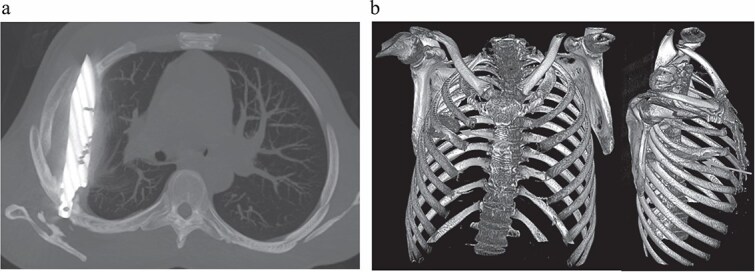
(a) CT chest maximum intensity projection (MIP) demonstrating a retained metallic foreign body (knife) traversing the right chest, with associated healed fractures. (b) 3D reconstructed CT chest image demonstrating the retained knife and associated skeletal injuries.
